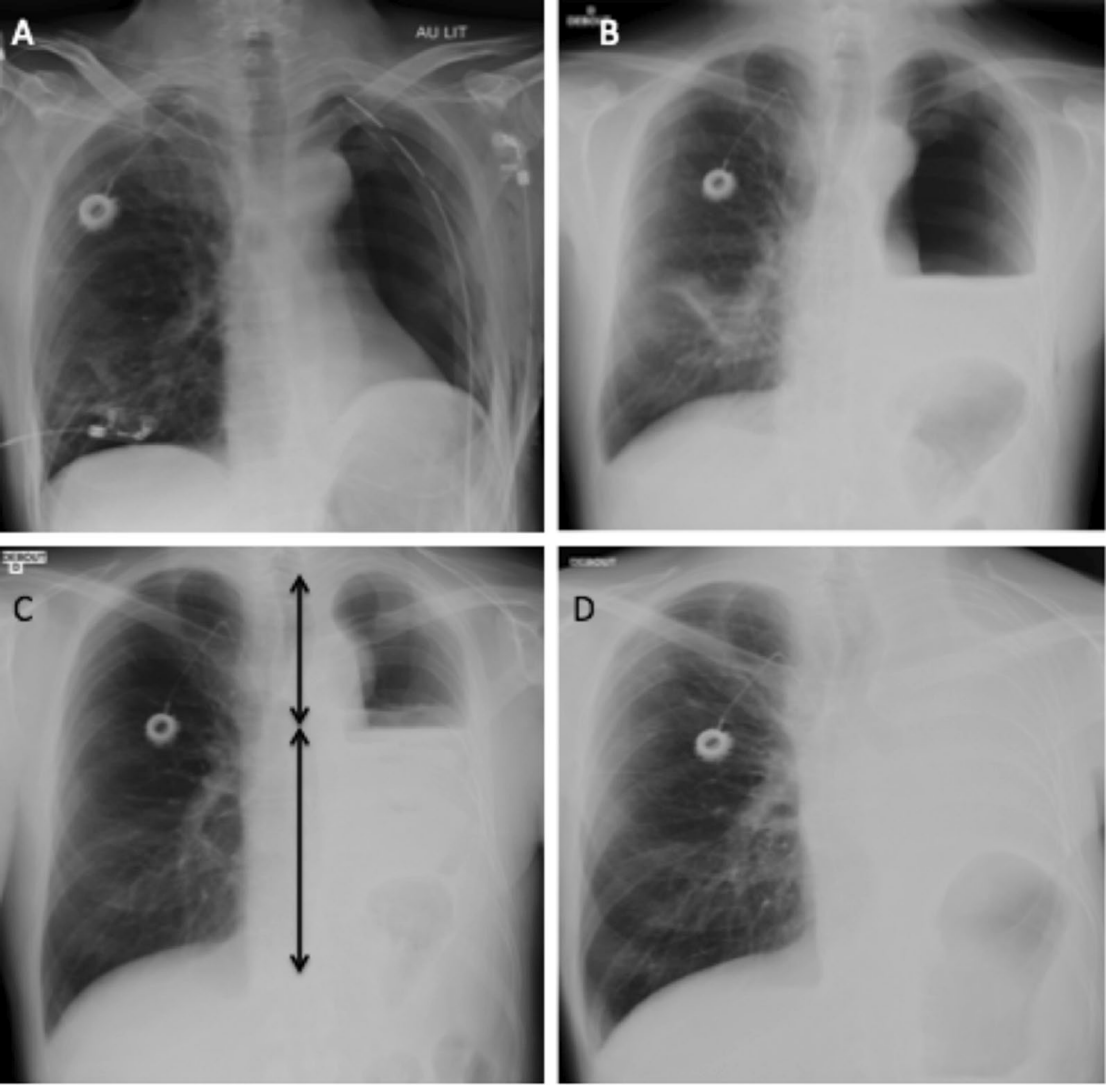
Pneumonectomy cavity evolution. A: First post-operative CXR in the hours following left pneumonectomy, with a chest tube in antero-apical position. B: Day 3 CXR with progressive filling of the pneumonectomy cavity. C: Day 7 CXR on which you can see that the air–fluid level reaches two-thirds of the pneumonectomy cavity. D: CXR after 2 months showing a complete filling of the cavityLater, the pulmonary arterial stump may be the site of an alluvial thrombus (Fig. 6), generally non-pathological if it is not progressive. A long bronchial stump may be the site of an accumulation of secretions of fluid like and sometimes tissular attenuation on unenhanced chest CT. In such case, contrast uptake would suggest local recurrence or superimposed infection. Mediastinal deviation on the side of the pneumonectomy is seen at variable degrees. Possible parietal sequelae that can consist of costal reshaping, herniation of the parietal fat at the site of thoracic wall resection, lymph node removal clips and possibly a high attenuation Goretex prosthesis can be present in case of diaphragmatic surgery.
